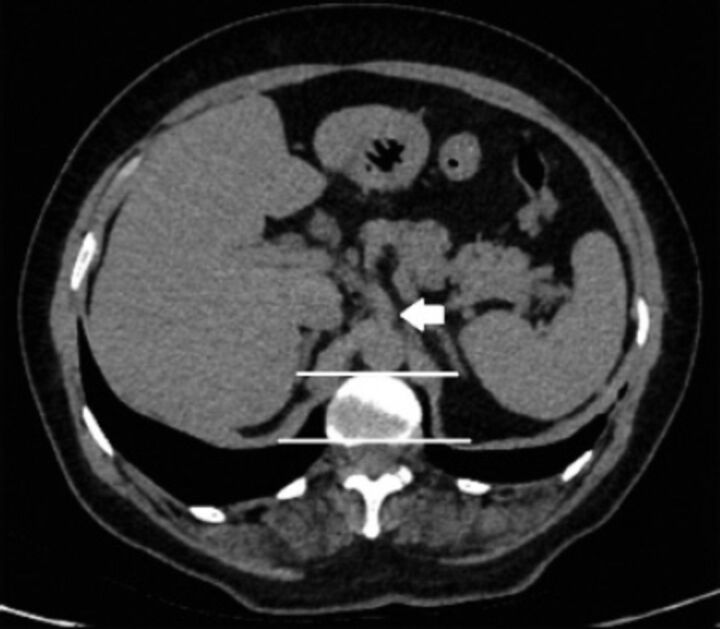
Points of diaphragm thickness measurements. Axial computed tomography scan at the celiac artery origin level (white arrow). Anterior and middle diaphragm thickness measurement points (white lines).
